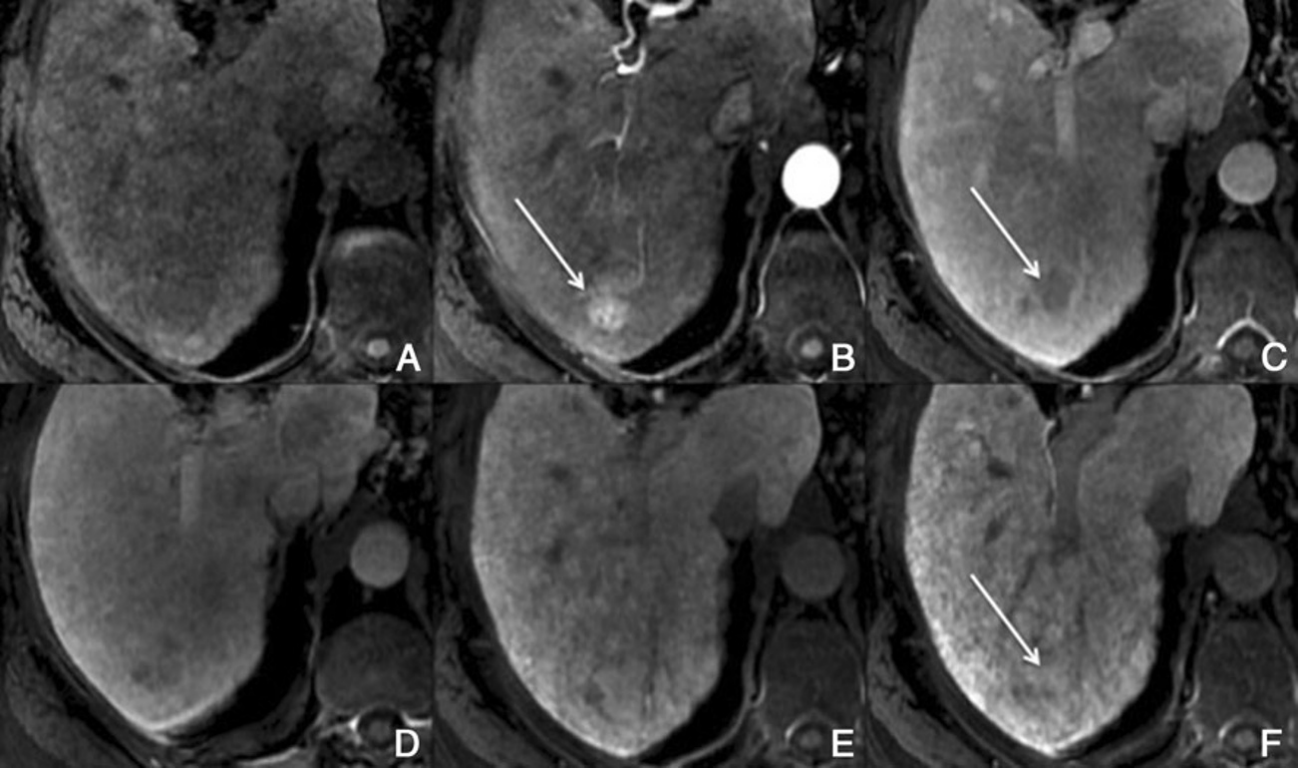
HCC in a 42-year-old woman with chronic liver disease. a Pre-contrast MRI, b arterial phase, c PV phase, d 5 min, e 10 min, f HCP. Hypervascular HCC, with washout in the PV phase and uptake of Gd-EOB-DTPA (*arrows*)
